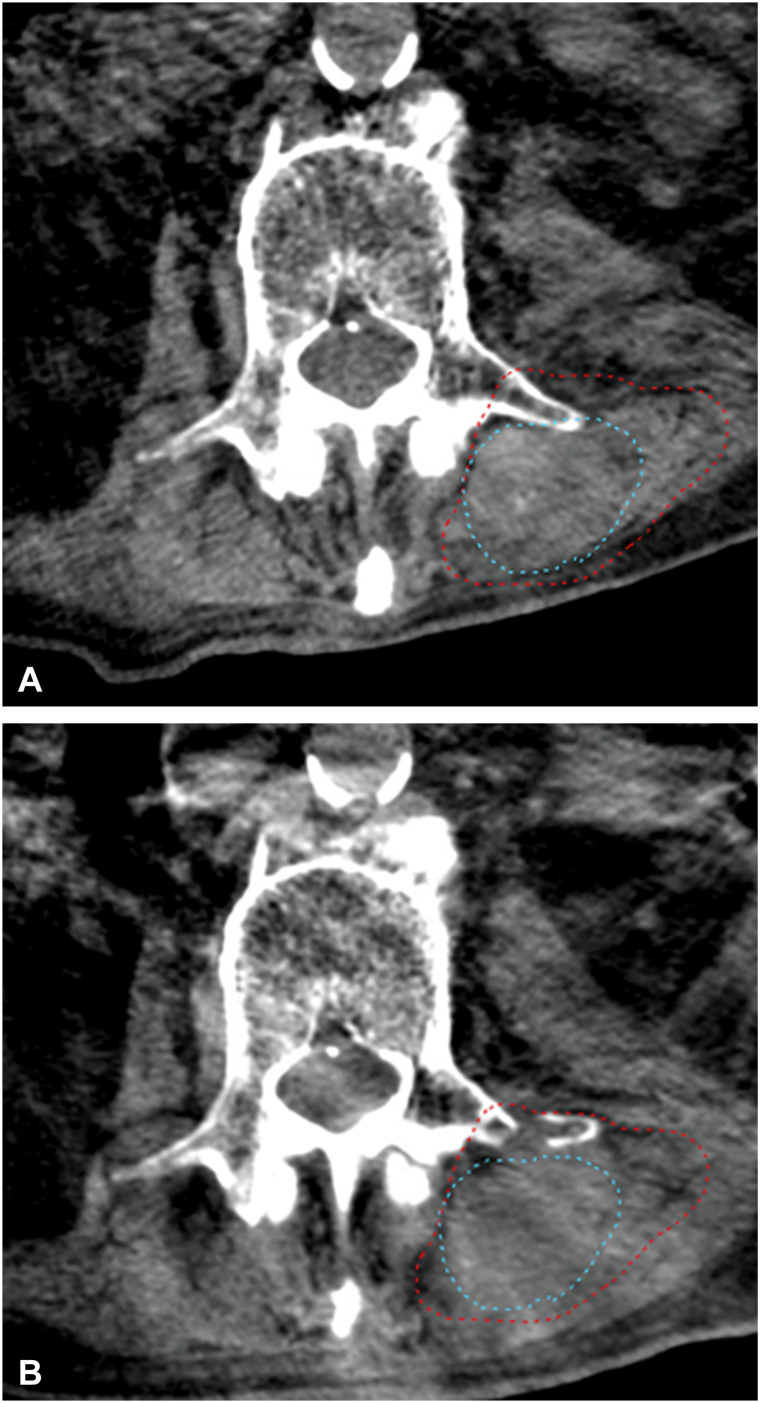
Axial cone beam CT of first (A) and fourth (B) day of SBRT. Disease progression is evident posteriorly where soft tissue density is extending beyond the adaptive GTV (*red*) and anteriorly where fracture of the left transverse process occured. *Blue* = initial GTV. *CT*, Computed tomography; *GTV*, gross tumor volume.
